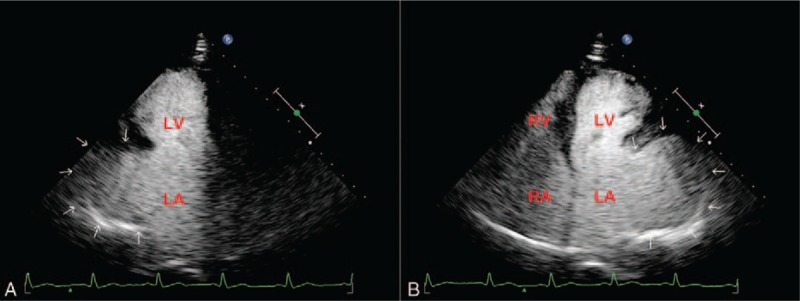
Contrast echocardiograms. Apical 3-chamber (A) and 4-chamber (B) views confirming this mass (arrow) communicating to the LA. LA = left atrium, LV = left ventricle, RA = right atrium, RV = right ventricle.
